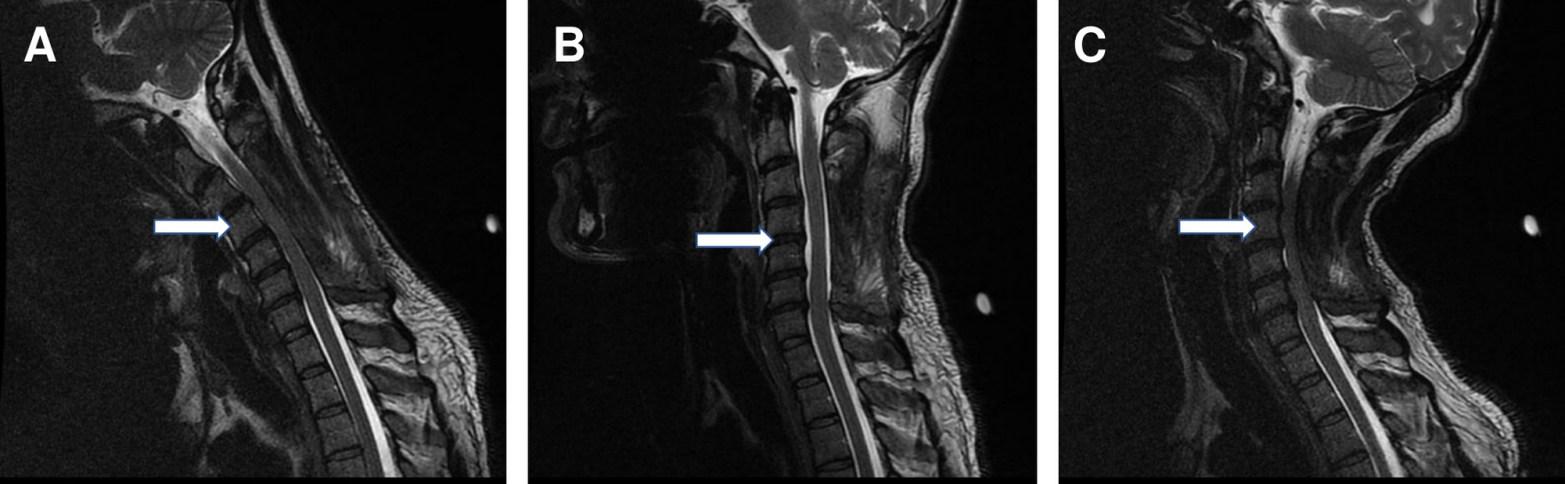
Flexion, Neutral, and Extension Magnetic Resonance Imaging Post-laminectomy Postoperative sagittal T2 magnetic resonance imaging in flexion (A), neutral (B), and extension (C) positions shows minor cervical spinal stenosis in a neutral position with more severe cervical spinal stenosis in flexion and extension positions.
